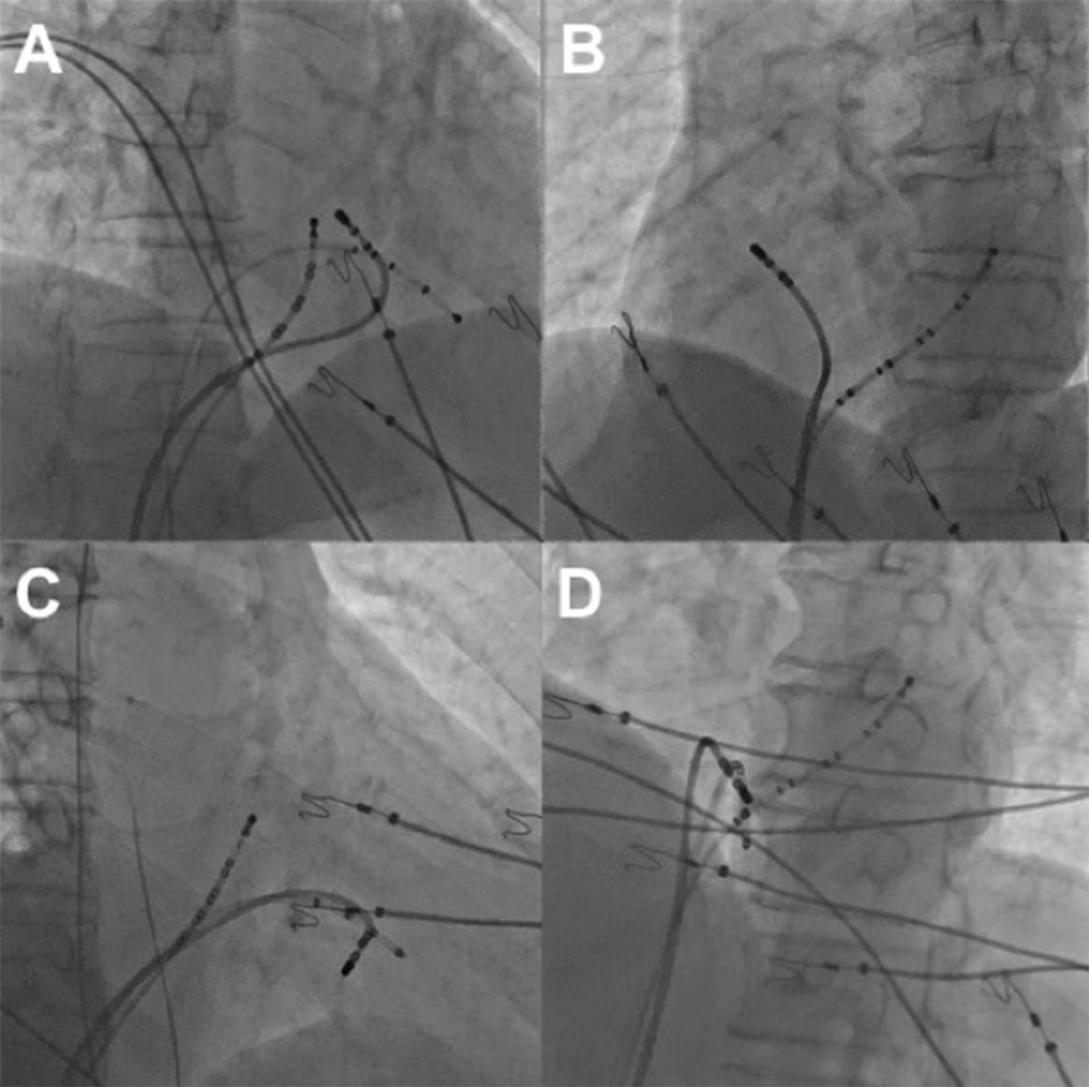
Successful ablation sites utilizing the reversed U-curve ablation technique (A) Right anterior oblique (RAO 30°) radiographic views of site at 11 o’clock; (B) Left anterior oblique (LAO 45°) radiographic views of site at 11 o’clock; (C) Right anterior oblique (RAO 30°) radiographic views of site at 5 o’clock; (D) Left anterior oblique (LAO 45°) radiographic views of site at 5 o’clock.
